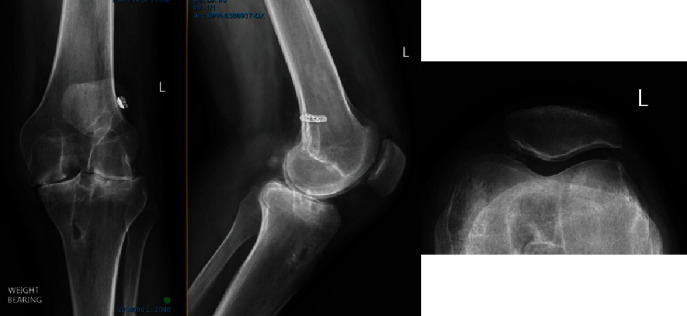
Radiograph in 2019 of BP's left knee. Note the significant interval change from 2016 images.
